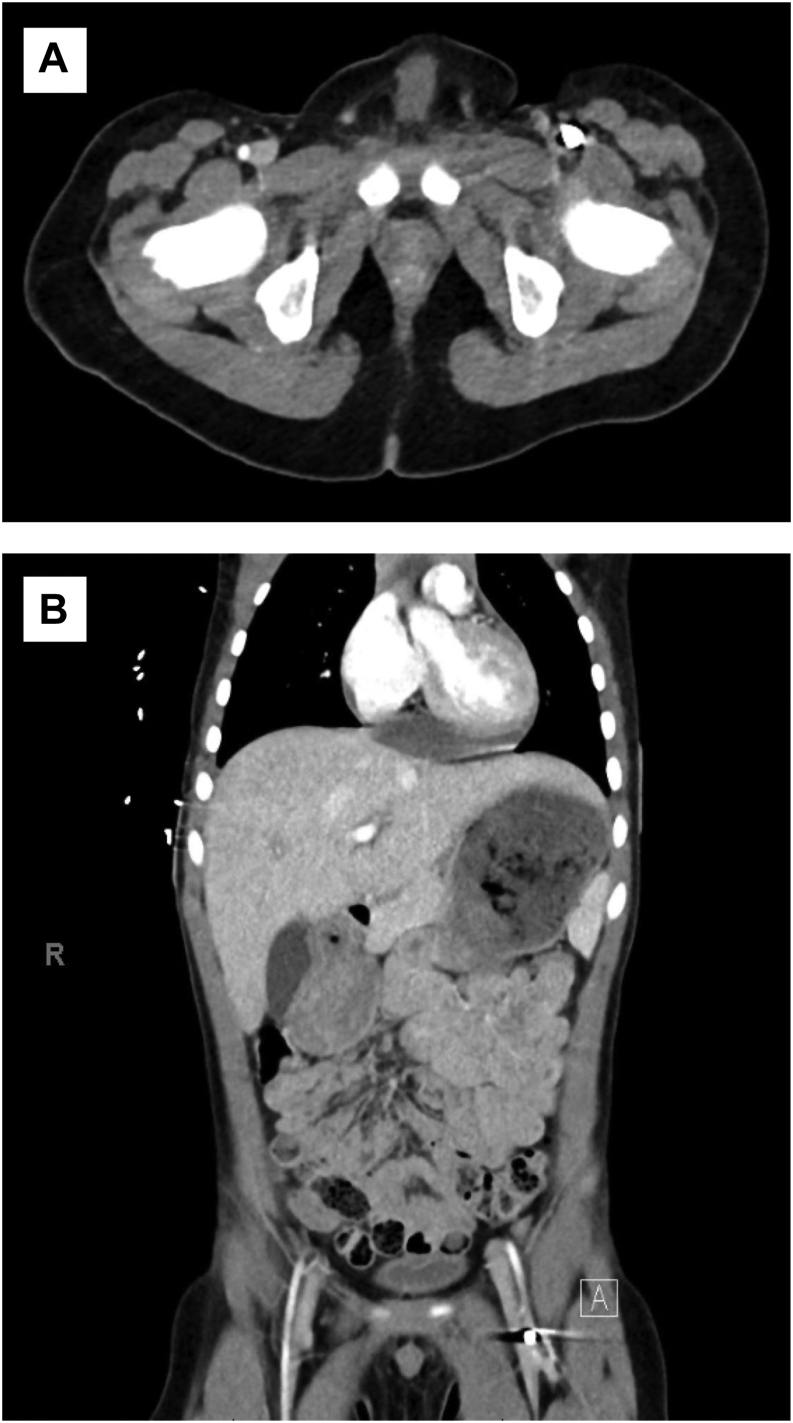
A, Axial view of computed tomography (CT) scan of the abdomen and pelvis with intravenous contrast (axial view) showing the pellet in the left common femoral artery (CFA). B, Coronal view of CT scan of the abdomen and pelvis with intravenous contrast showing the pellet at the bifurcation of the left CFA to the profunda femoris artery and superficial femoral artery.
